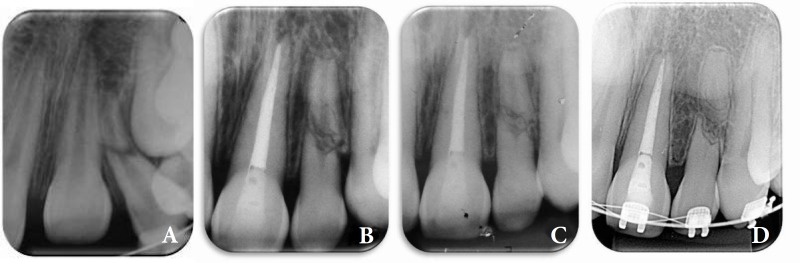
A) *Initial periapical radiography demonstrating the intrusion of the left maxillary central incisor and the horizontal fractures in the middle third of the left maxillary lateral incisor; *B)* Periapical radiographs with 2 years follow-up; *C)* Periapical radiographs after 5 years follow-up; *D)* Periapical radiographs with 8 years follow-up
